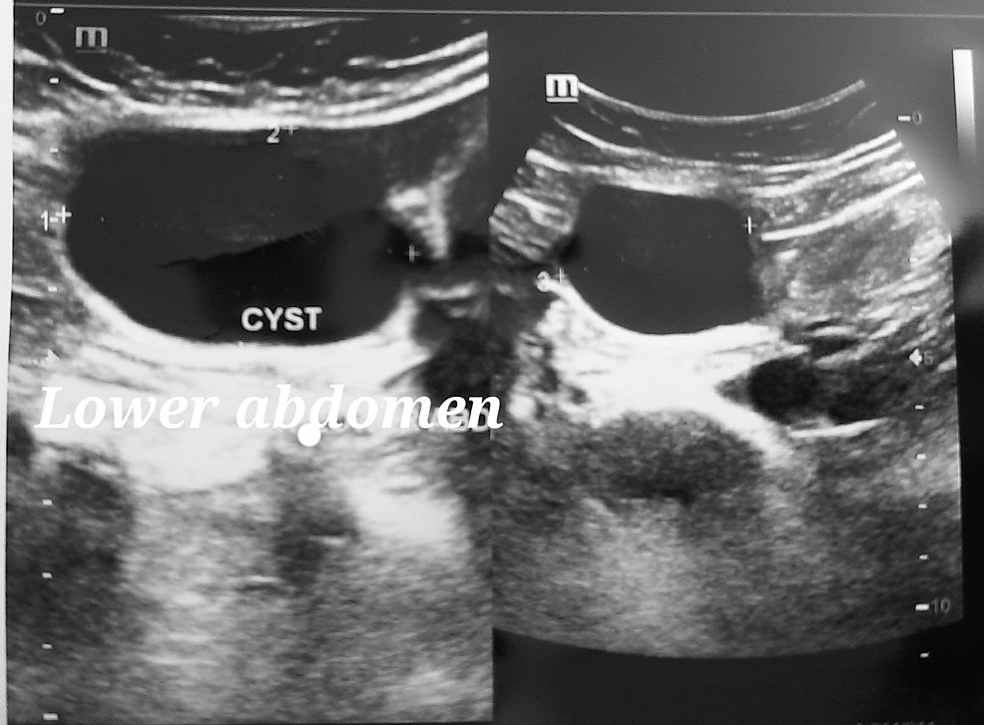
Ultrasonography showing a cystic mass in the lower abdomen
